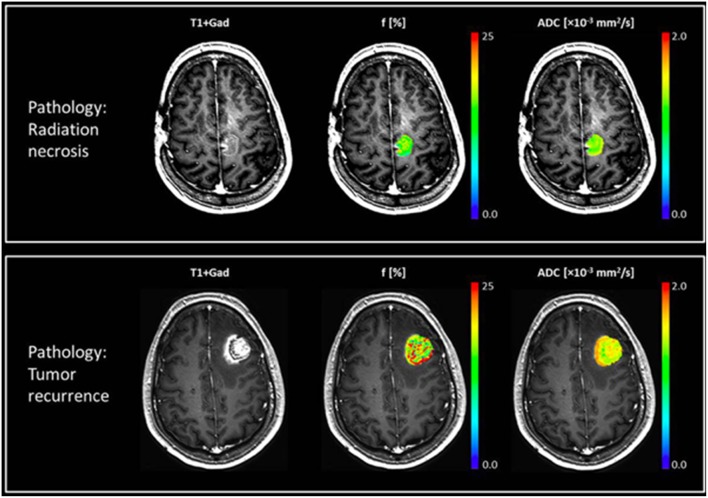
Two patients with brain metastases presenting with enlarging enhancing mass after treatment with SRS. Top row shows a case of radiation necrosis, and bottom row shows a case of recurrent tumor. Post-Gd T_1w_-MRI (left), perfusion fraction *f*-map (middle), and ADC map (right) are shown for both cases, where the patient with radiation necrosis exhibits a uniformly low perfusion fraction while the patient with recurrent tumor has more heterogeneous maps with a higher perfusion fraction. In these two case the ADC values were similar but slightly higher for radiation necrosis. Reproduced, with permission from Detsky et al. (46).
